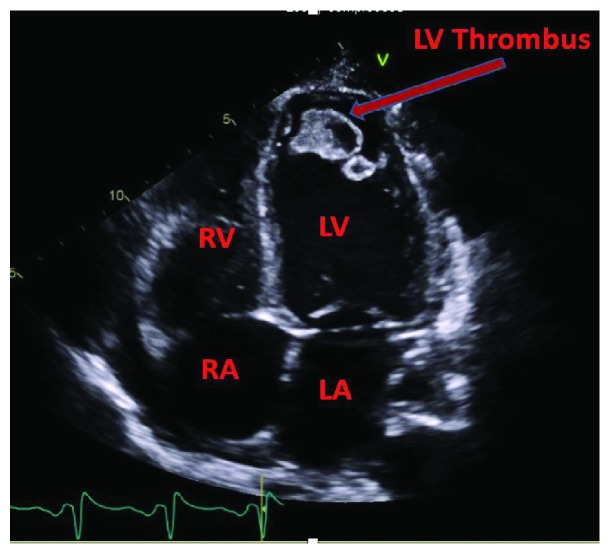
Apical four-chamber view showing LV thrombus. LA: left atrium; RA: right atrium; RV: right ventricle; LV: left ventricle.
